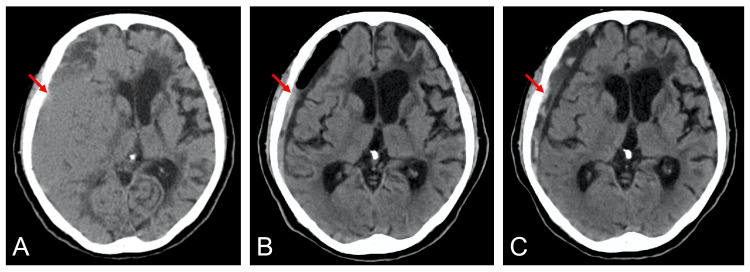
Head computed tomography Head computed tomography showing preoperative chronic subdural hematoma (A), and that on postoperative day 1 (B) and postoperative day 3 (C) (arrows)
